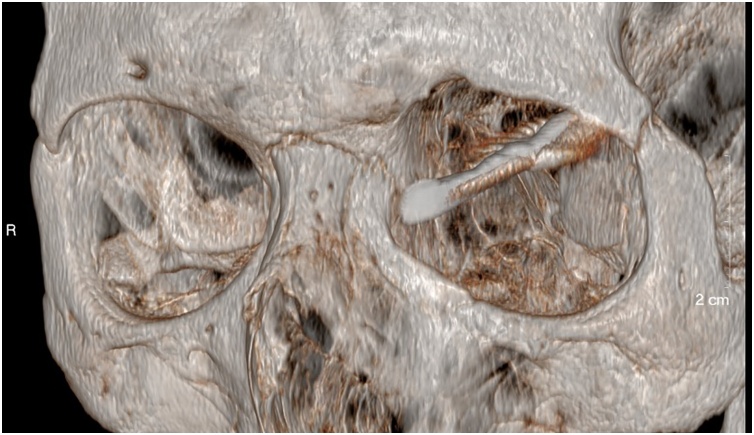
3D reconstruction of orbit CT-Scan.
